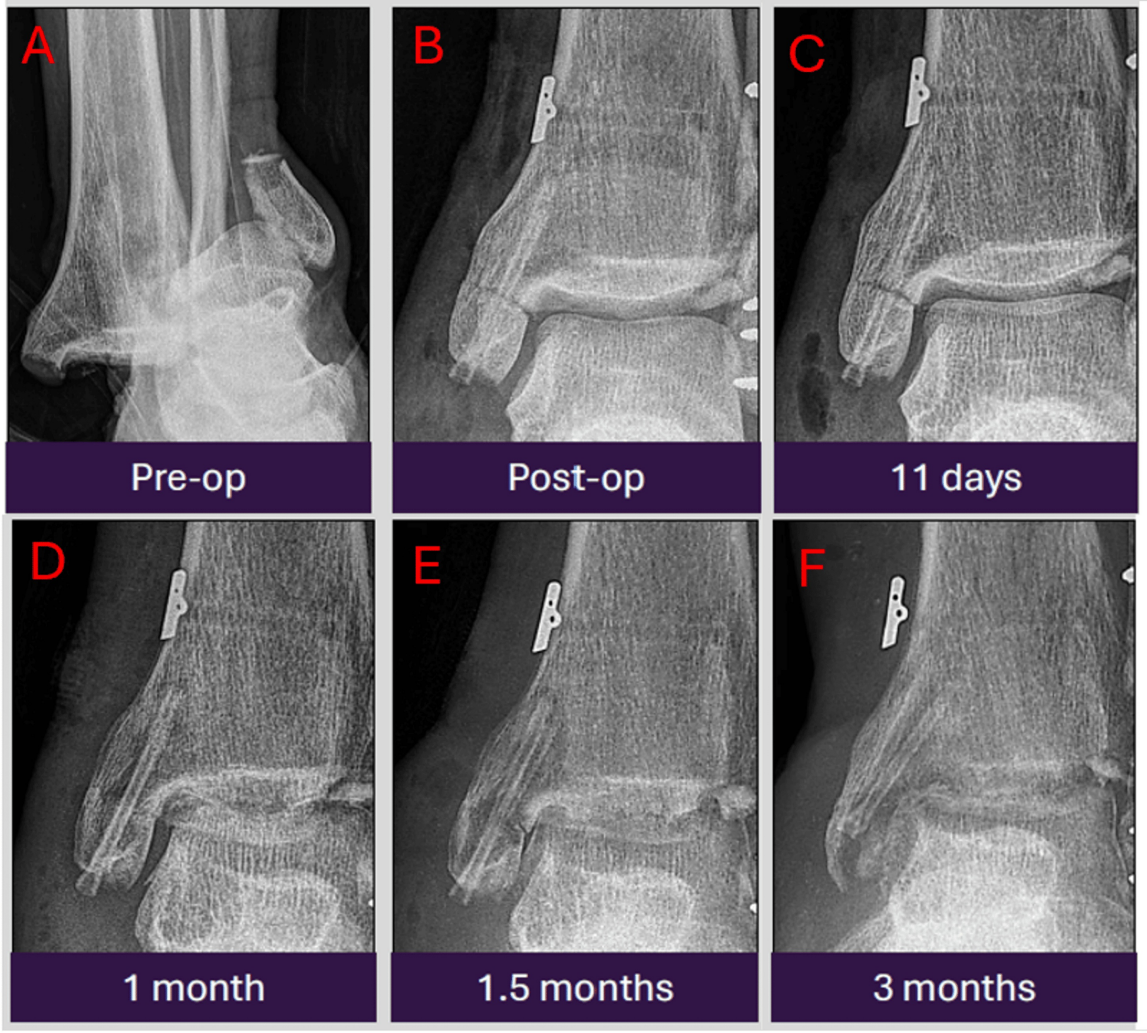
Serial antero-posterior radiographic images from pre-operation to three months post-operation showing peri-implant radiolucencies which are considered a normal feature of biocorrosion in the absence of clinical deterioration. The radiographs were obtained at pre-operation (A), immediately post-operation (B), 11 days (C), one month (D), 1.5 months (E), and three months (F) post-operation.
